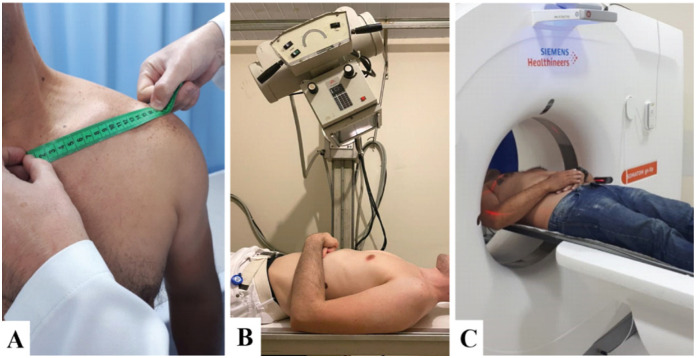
Methods of measuring clavicular length. (A) Physical examination using the metric tape; (B) Radiographic examination with anteroposterior incidence with a caudocranial axial projection of 20°; (C) Examination by computed tomography.
